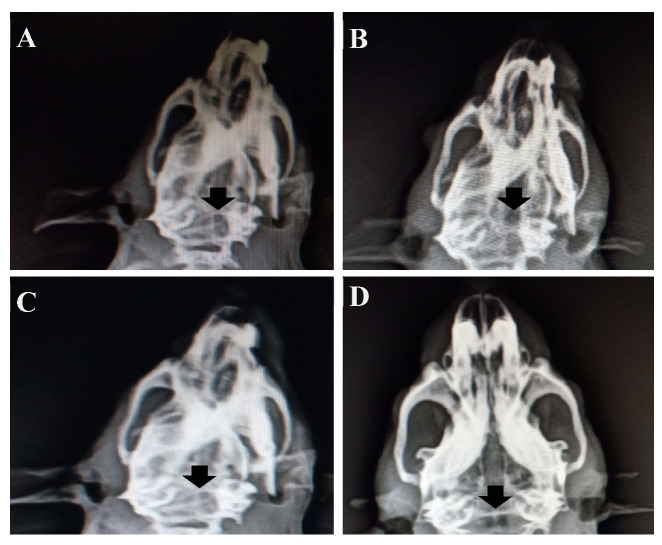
X-ray images of (A) without implantation at 4 weeks (B) HAP-HPC-PLA at 4 weeks (C) without implantation at 8 weeks (D) HAP-HPC-PLA at 8 weeks.
